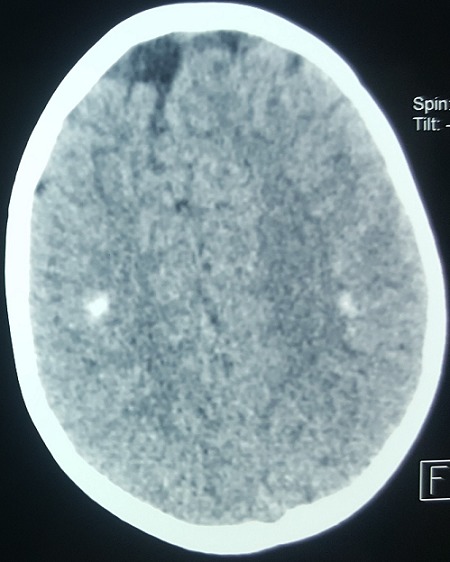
Cranial CT scan demonstrating bilateral supra-tentorial and sub-ependymal and cortical calcifications
